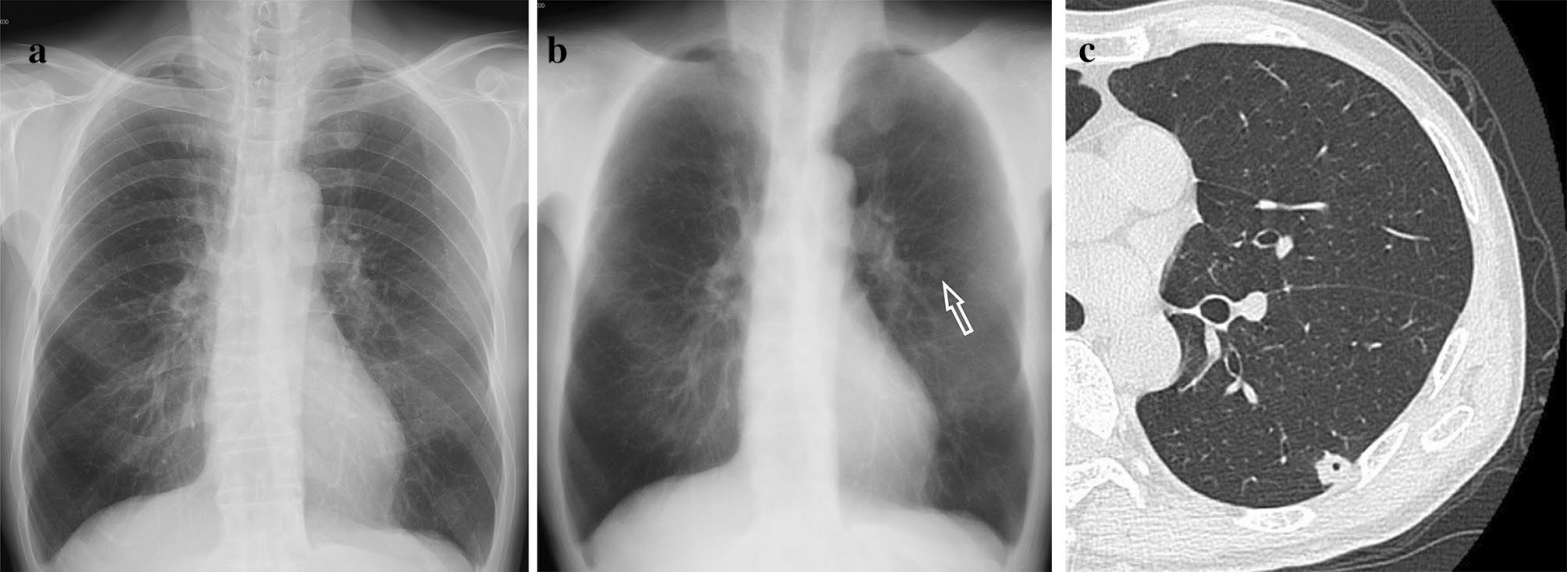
Male, 70 s, squamous cell carcinoma, 10 mm. a No abnormal findings were observed on conventional radiography. b The soft tissue image showed a nodular shadow in the left middle lung field (arrow). c CT showed a nodular shadow in the left lower lobe
